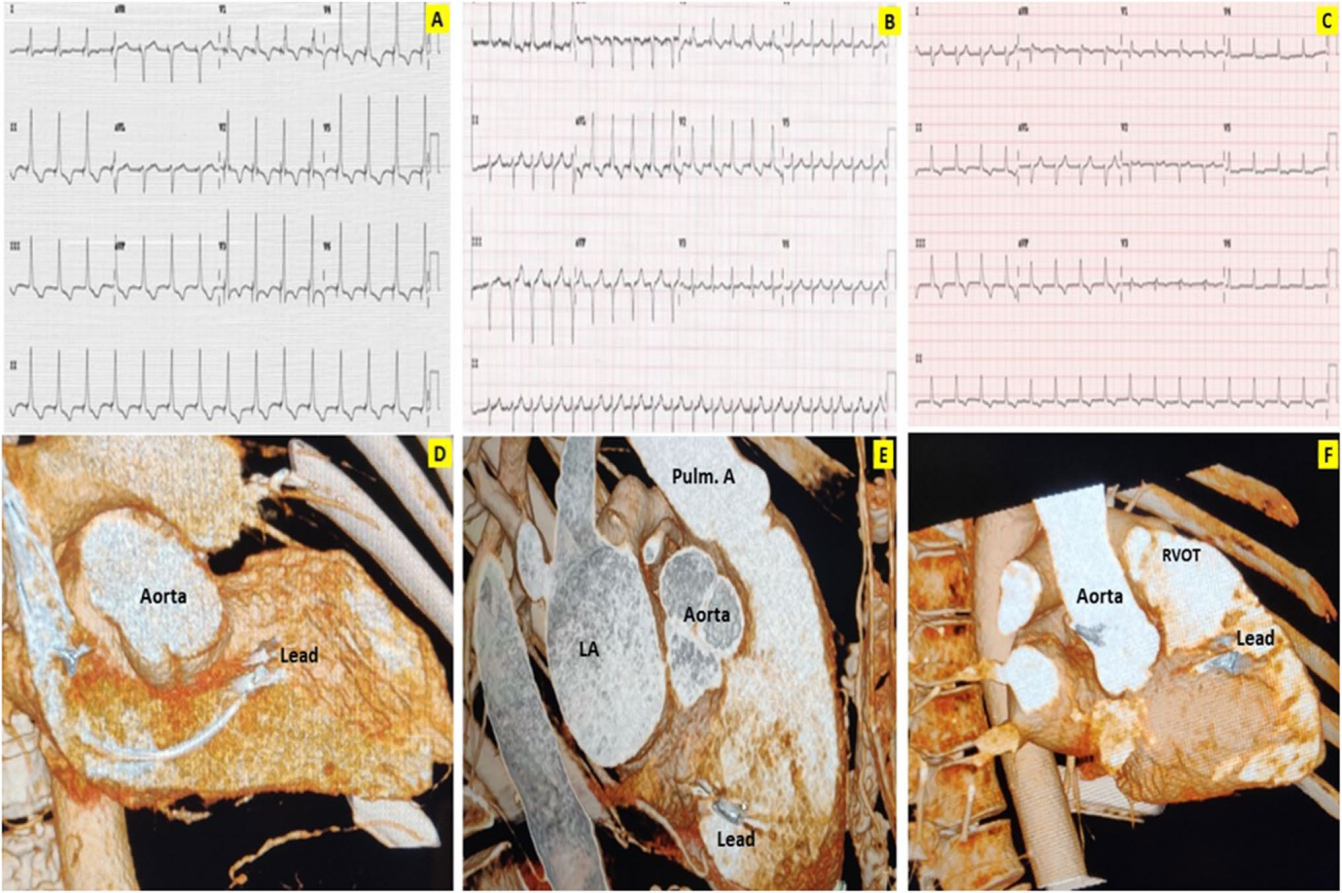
Left bundle branch pacing. A shows main left bundle capture, B shows left posterior fascicle (LPF) capture, C shows left anterior fascicle (LAF) capture. (See Appendix 3 for larger images). Note narrow QRS and qR patterns in V1 in all ECGs. A shows normal QRS axis suggesting main left bundle capture. B shows the left axis deviation of QRS and C shows the right axis deviation of QRS suggesting capture of LPF and LAF respectively. D–F show corresponding CT images of the pacing lead location confirming existing understanding of the location of fascicles of the left bundle
